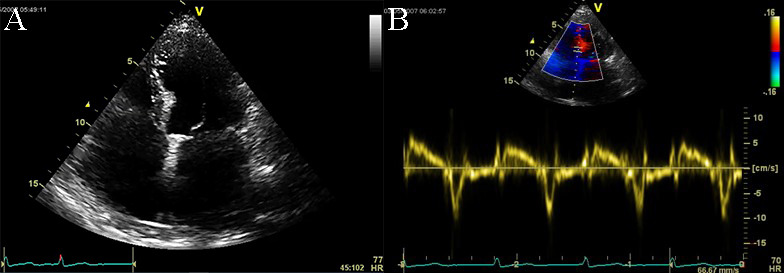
Echocardiographic aspect of RCM. (A) Apical 4c view of an
